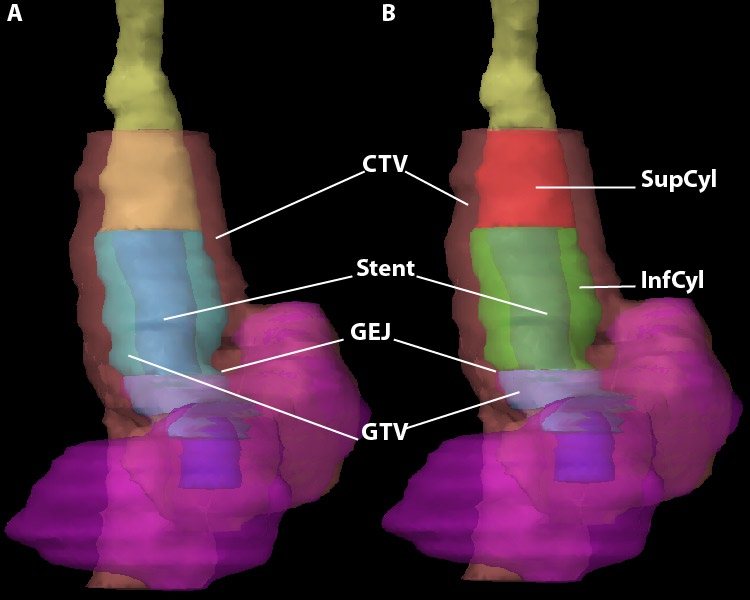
Coronal volume 3D reconstruction (a) showing the esophagus (yellow), with a self‐expanding stent (SES blue) extending past the gastroesophageal junction (GEJ) into the stomach (magenta) with the GTV (cyan) and CTV (pink) visible; Coronal volume 3D reconstruction (b) now showing post‐stent SupCyl and InfCyl structures (red and green, respectively). When fusing the pre‐stent to the post‐stent CT scans, priority was given to overlaying the SupCyl and InfCyl volumes of each scan, especially in the craniocaudal direction.
